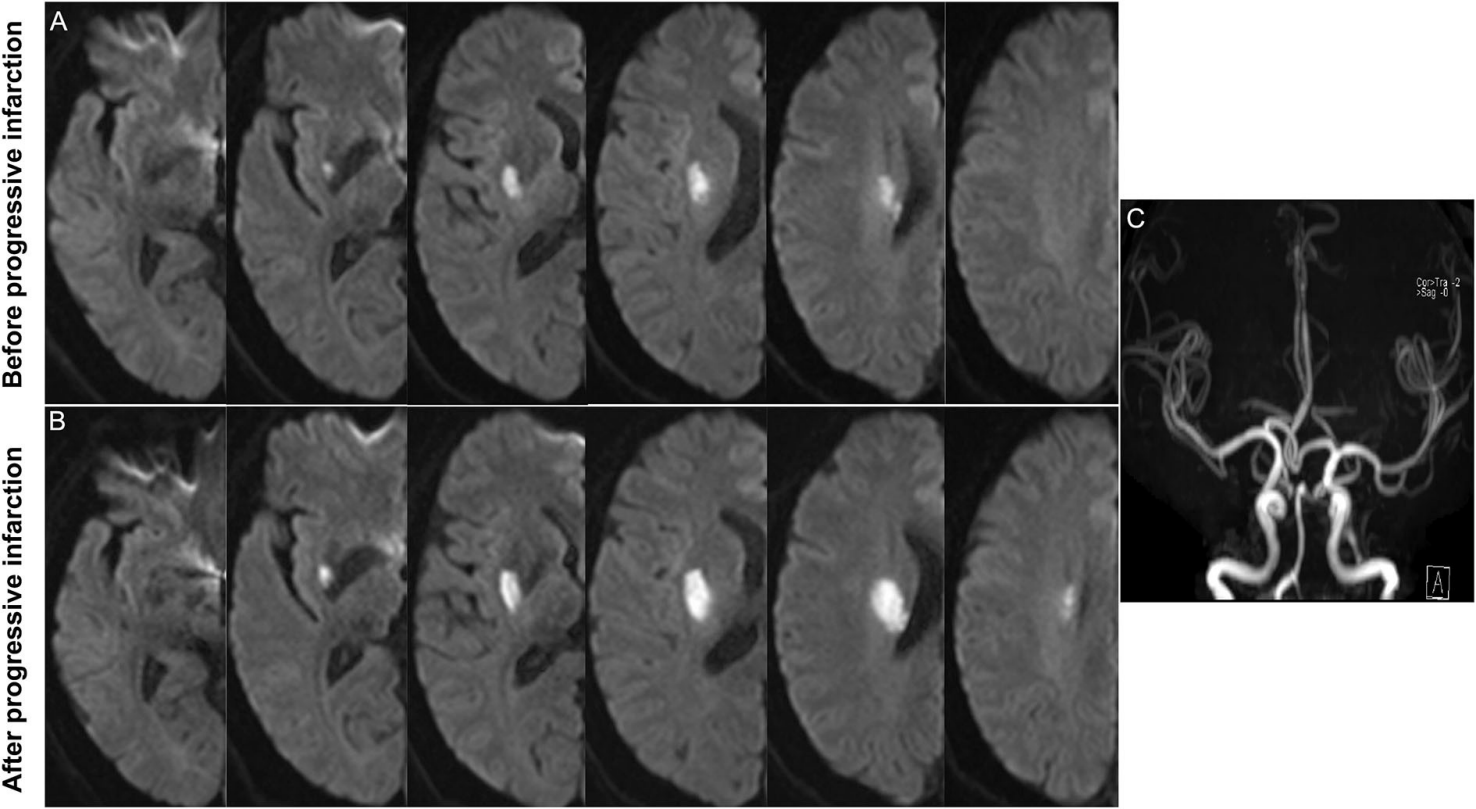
Representative case of progressive infarction. (A) Initial axial DWI indicated the infarct slice number was ≥ 3. (B) Axial DWI showed the growth of infarction. (C) MRA showed no obvious etiologies (vasculitis, dissection, Moyamoya disease) that led to ischemic stroke.
